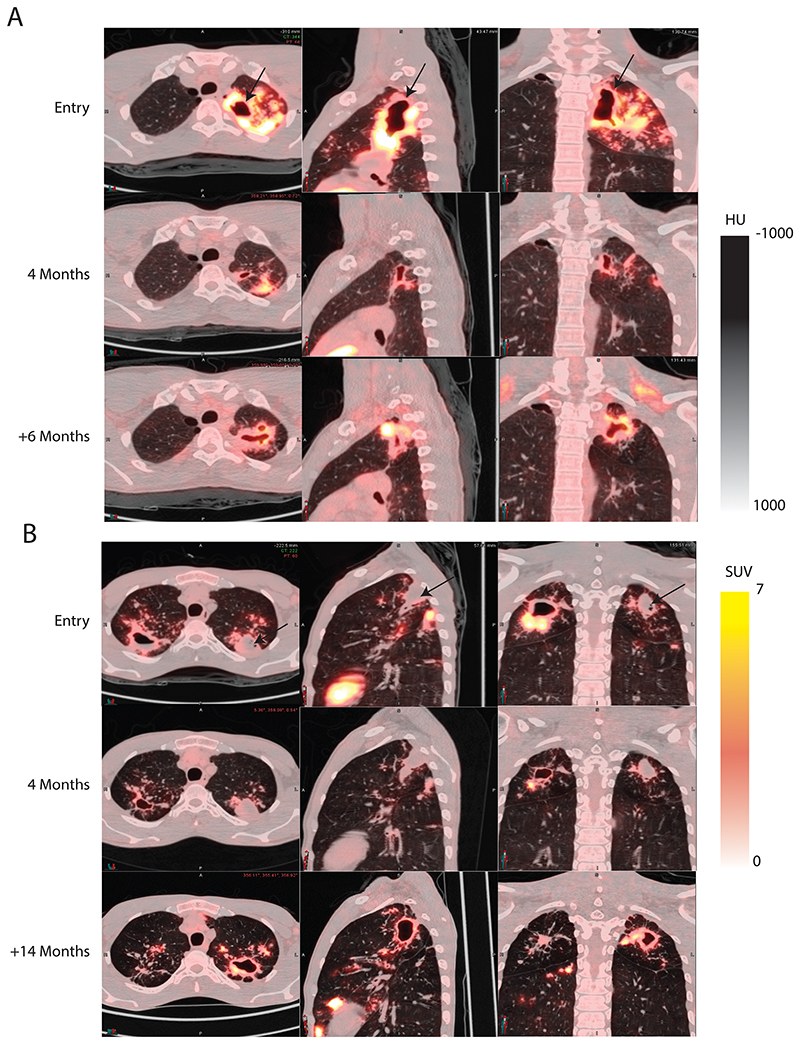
TB relapse involves baseline lung cavities. (A) Participant A19 in Arm A presented with a large left apical cavity on the PET/CT imaging scan(arrow top row), which appeared to have mostly resolved after four months of treatment. Six months after completing treatment the participant became sputum culture positive again with the same isolate and with a large left apical cavity in the same region of the lung. (B) Participant A9 presented with bilateral disease with a left upper “dirty” cavity (arrow on entry scans) with cold central necrotic material and only slight liquefaction. After four months of treatment the right apical cavity showed substantial response, whereas the cold left side lesion appeared less active. At 14 months from baseline the participant experienced relapse and became sputum culture positive with an identical isolate, and the necrotic left apical lesion appeared to be an active thick-walled cavity.
